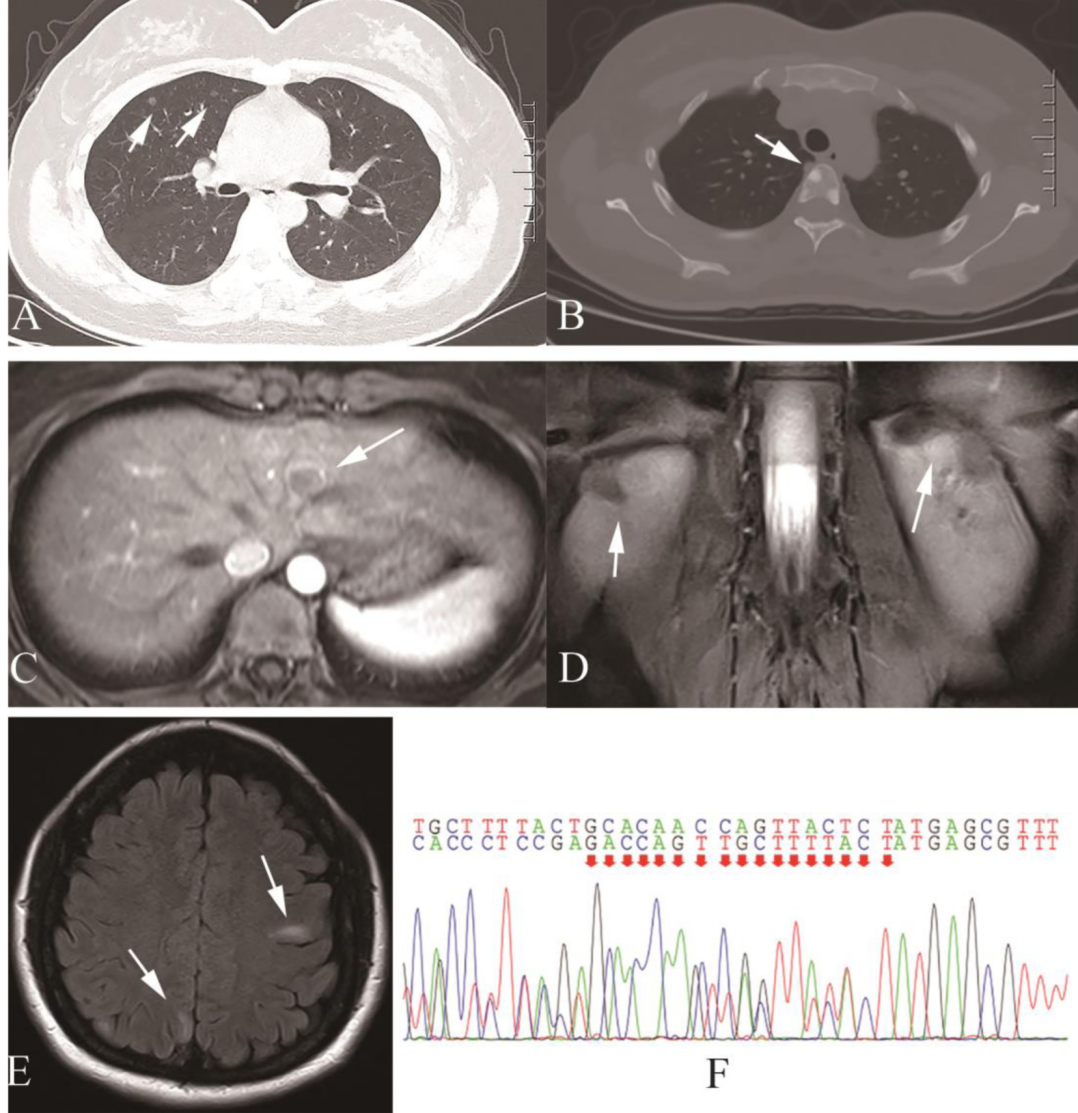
(A) Chest computed tomography (CT) revealed multifocal micronodular pneumocyte hyperplasia. (B) Thoracic spine CT revealed sclerotic bone lesions. (C, D) Abdomen magnetic resonance imaging (MRI) revealed angiomyolipoma on both kidneys and the left liver lobe. (E) Fluid attenuated inversion recovery brain MRI revealed cortical tubers. (F) Chromatogram confirmation indicated c.2094_2110del (p.His699Ter) mutation in *TSC1.*
